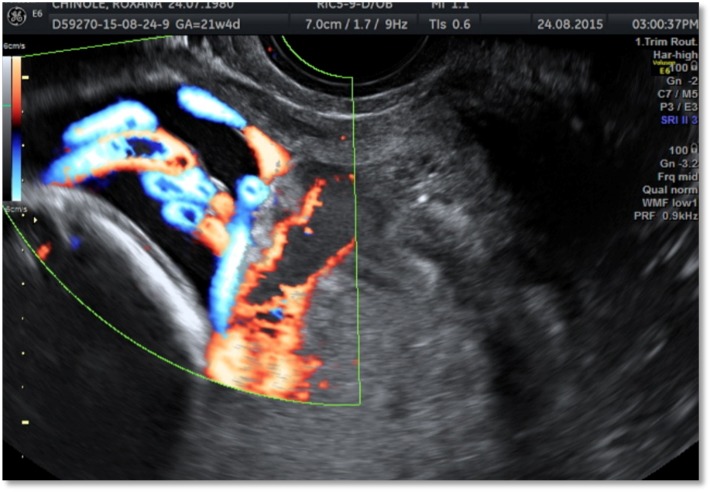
Case 2: HD flow transvaginal Doppler examination; velamentous insertion of the low-lying placenta with vasa praevia
